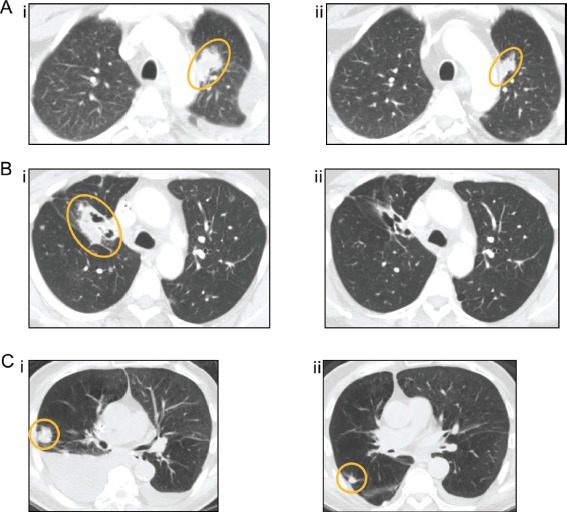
Computed tomography (CT) images of the three patients with the greatest tumor reduction (A) patient #37 (EGFR mutation not done, smoker), who achieved a PR (59% decrease), at baseline (i) and 32 weeks (ii), (B) patient #197 (EGFR L858R, G873E and PIK3CA E542K mutations, nonsmoker) who achieved a PR (55% decrease), at baseline (i) and 20 weeks (ii), and (C) patient #200 (EGFR wild-type, smoker), who achieved an unconfirmed PR (48% decrease), at baseline (i) and 8 weeks (ii).
